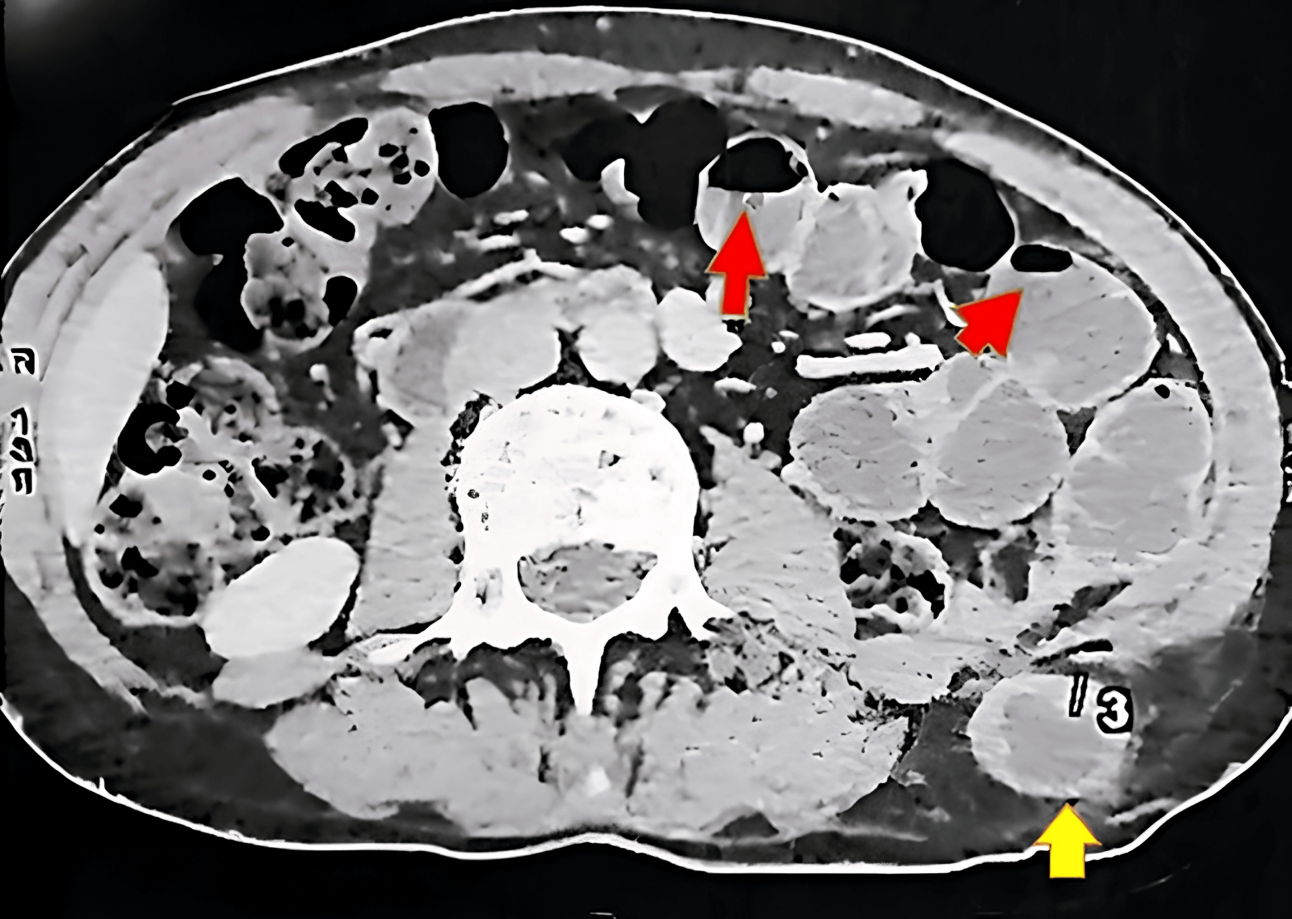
Axial CT scan demonstrating air-fluid levels due to bowel obstruction secondary to an incarcerated lumbar hernia. Dilated small bowel loops with air-fluid levels (red arrows) indicate mechanical obstruction. The incarcerated bowel segment in the left lumbar hernia (yellow arrow) shows a 30 mm loop dilatation with 8 mm wall thickening (3) from compromised perfusion.
